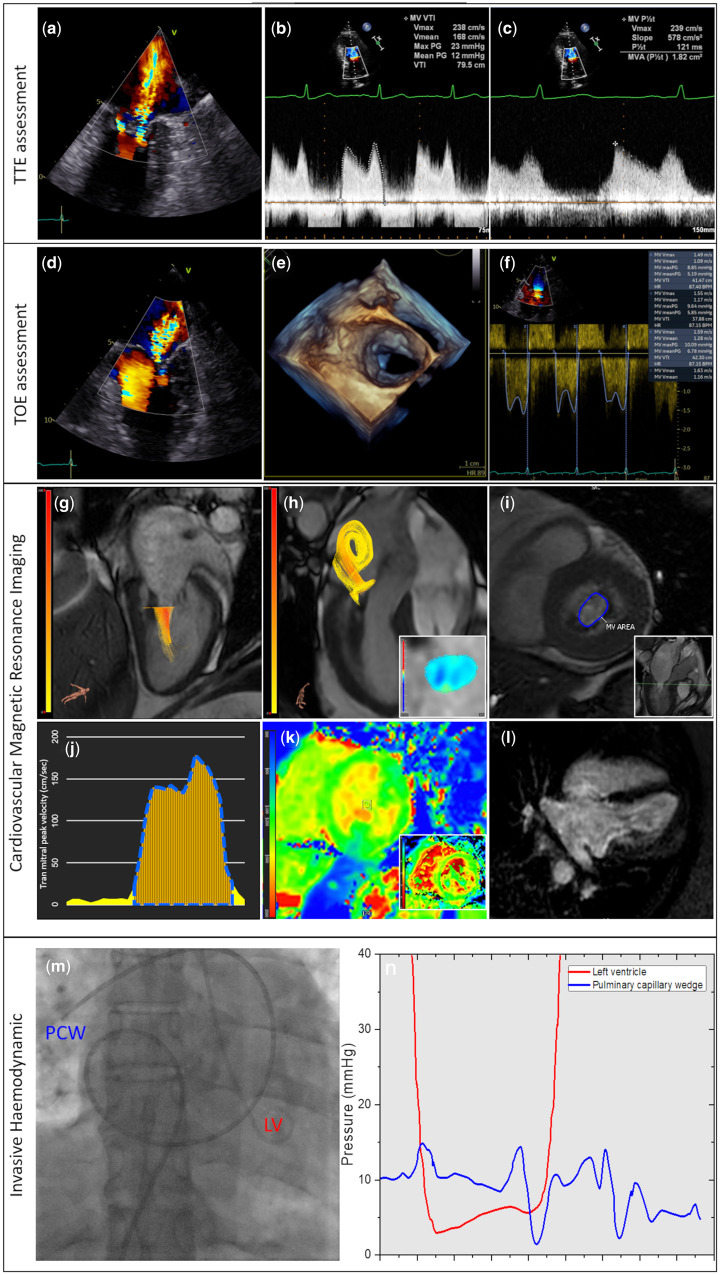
Multi-modality assessment of mixed mitral valve disease. Transthoracic echocardiography: Turbulent mitral regurgitation demonstrated in the apical two chamber with dilated left atrium (*A*). Velocity time integral-derived mean pressure gradient through the mitral valve (*B*) was 12 mmHg and the pressure half-time (*C*) estimated the mitral valve area to be 1.82 cm^2^. On transoesophageal echocardiography, severe mitral regurgitation is seen in two-chamber (*D*) and on three-dimensional reconstruction of the mitral valve (*E*) the degree of mitral stenosis does not appear to be severe. On velocity time integral (*F*), the mean pressure gradient was 6 mmHg. On four-dimensional flow cardiovascular magnetic resonance, the peak mitral inflow velocity was traced throughout the diastole using plane with maximum velocity (*G*) and during systole (*H*), a reformatted plane was generated perpendicular to the mitral regurgitation jet in the left atrium (*H*). On short-axis cine, mitral valve area was 2.1 cm^2^ at the tips of mitral valve (*I*). The mean pressure gradient for mitral inflow was 6 mmHg (*J*). T1-mapping (*K*) and extracellular mapping demonstrated normal myocardium. There was no evidence of left ventricular scar on late gadolinium enhancement (*L*). Invasive haemodynamic assessment was done using a multipurpose catheter placed for the capillary wedge pressure and a pigtail catheter within the left ventricle to allow recording of simultaneous pressures (*M*). The simultaneous pressure tracings of left ventricle and pulmonary capillary wedge are demonstrated (*N*).
